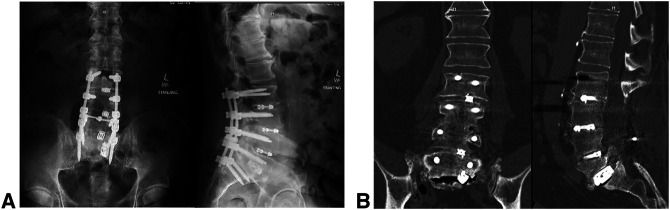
A, AP and lateral radiographs and (B) coronal and sagittal views of the lumbar spine showing L2-S1 instrumented fusion with L5-S1 pseudarthrosis and a vacuum disk space with cage subsidence.
